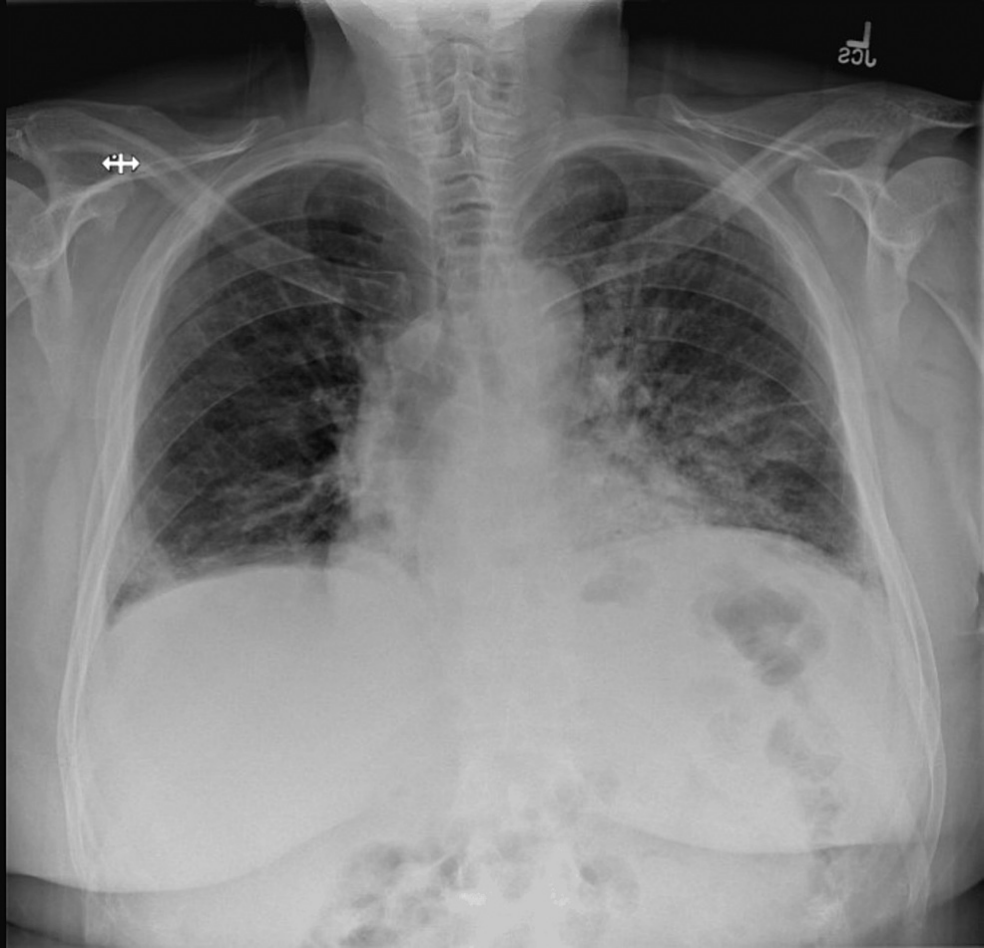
Chest X-ray on admission showed more perihilar interstitial prominence on the left than on the right.
